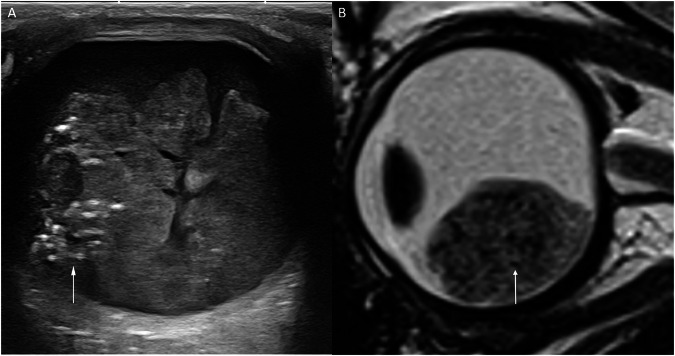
Microcalcifications in retinoblastoma: A Ultrasound image obtained using a high-frequency (up to 22 MHz) linear probe, showing a heterogeneous hyperechoic intraocular mass arising from the retina, with highly reflective shadowing (arrow) suggestive of calcification, and nearly pathognomonic for retinoblastoma. B Sagittal T2-weighted spin echo MR image revealing a solid intraocular mass containing multiple hypointense spots, compatible with microcalcifications
